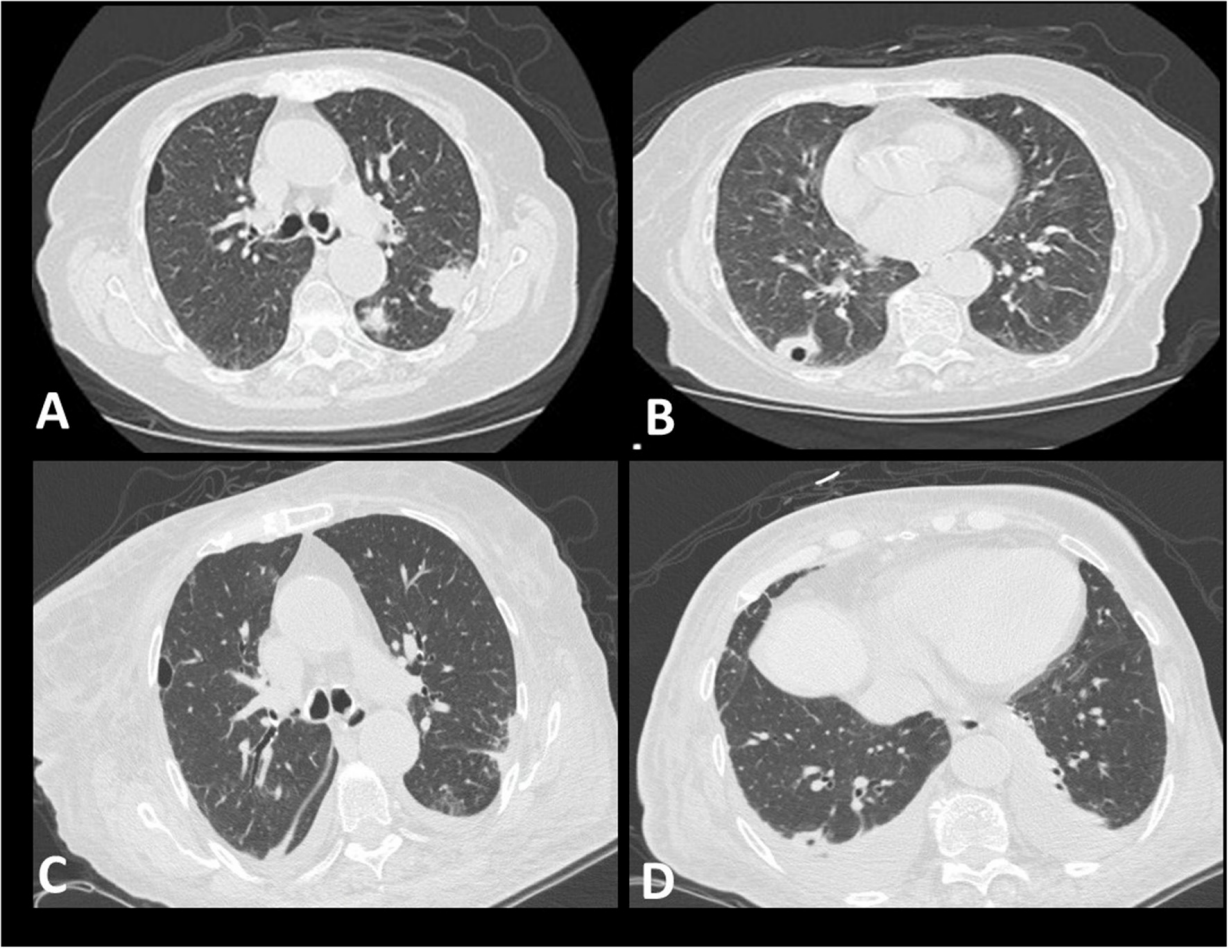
Radiological features. (A,B) showing multifocal consolidative opacities in the left upper lobe and one cavitary lesion in the right lower lobe (A,B) (day 1). Subtle ground glass opacities across the bronchovascular bundle can also be seen (B). Mild improvement of the radiographic appearance of the lesions is evident following treatment with pulses of methylprednisolone and cyclophosphamide (C). Small bilateral pleural effusions are shown, indicating possible fluid overload (D).
